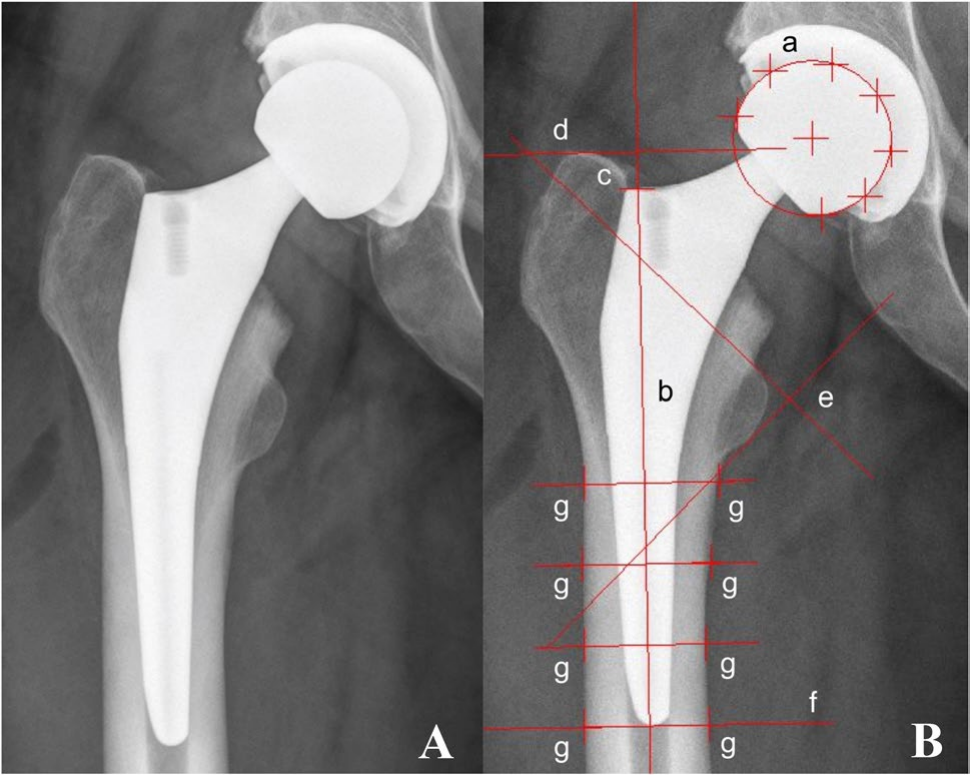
Anterior to posterior X-rays showing an Accolade II stem (A) and with EBRA-FCA references (B) a head points b stem axis c stem shoulder d major trochanter line e minor trochanter lines f tip-of-stem line g points at femoral bone contour
